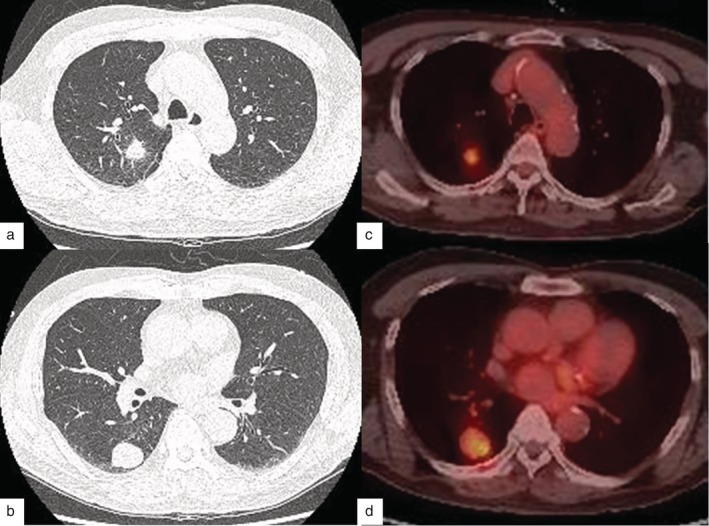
Chest computed tomography scans showed (a) an irregular nodular consolidation with mild spiculation and fissural retraction in the posterior segment of the right upper lobe (RUL) and (b) a well‐marginated mass in the superior segment of the right lower lobe (RLL). The positron emission tomography scan showed increased uptake (c) in the RUL (maximum standardized uptake value [SUVmax] 4.6) and (d) RLL (SUVmax 5.9).
